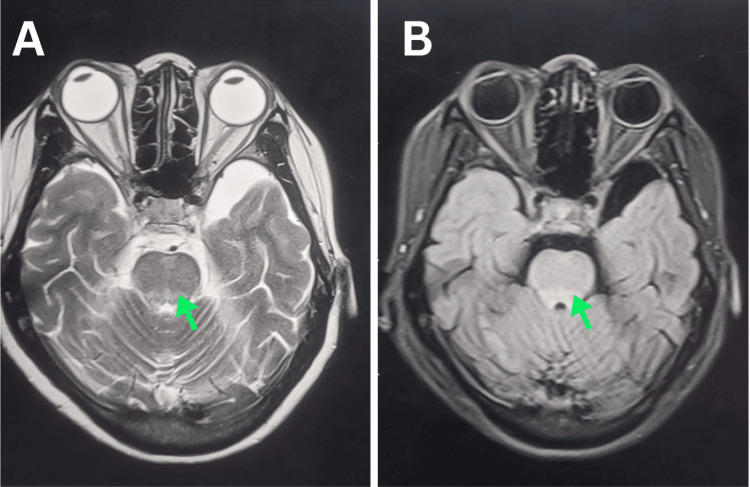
Axial T2 (A) and FLAIR (B) MRI brain showing high signal intensity at the level of the midbrain.
